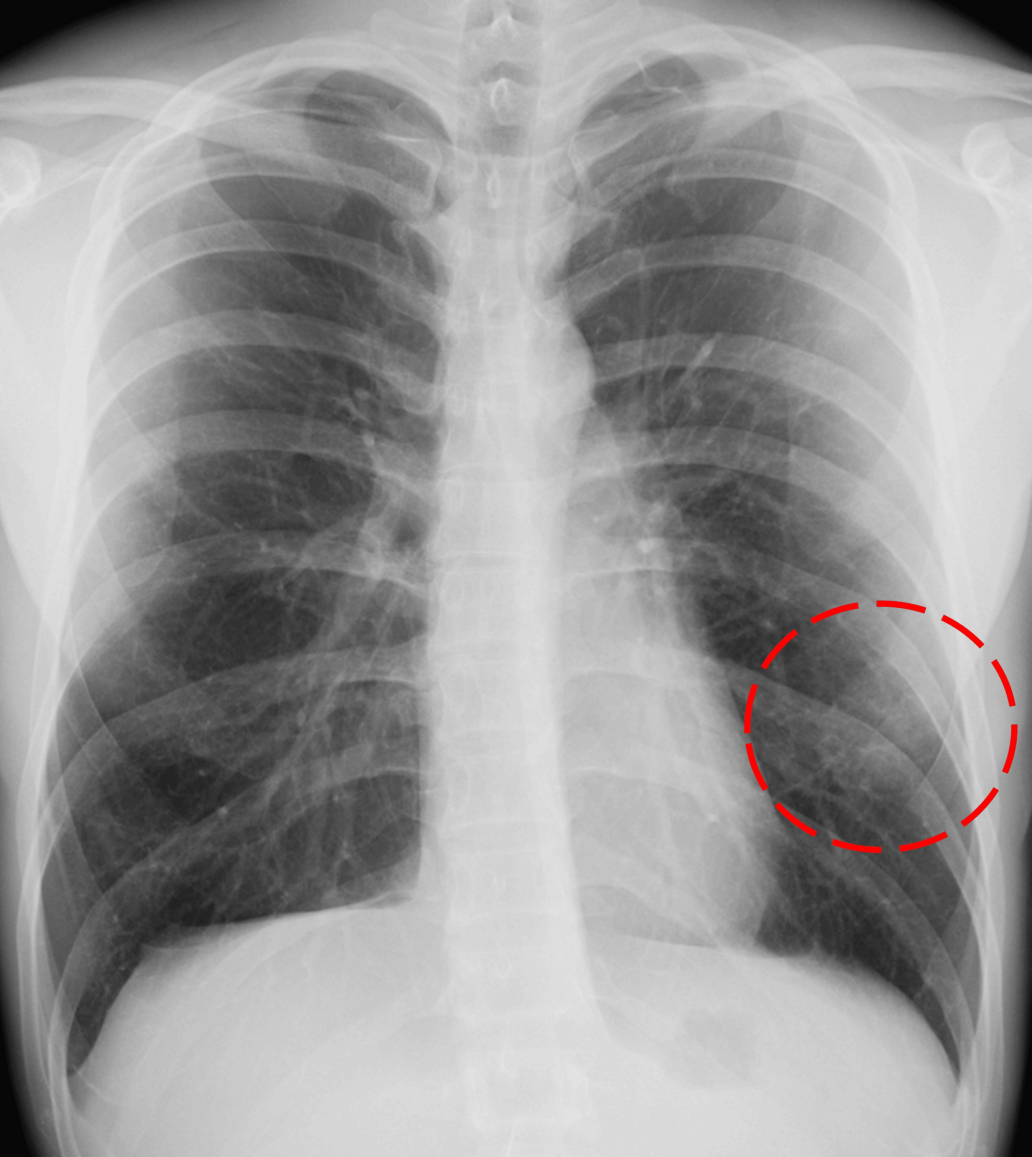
Chest radiograph The chest X-ray showed an ill‑defined focal opacity in the lower left lung field.
